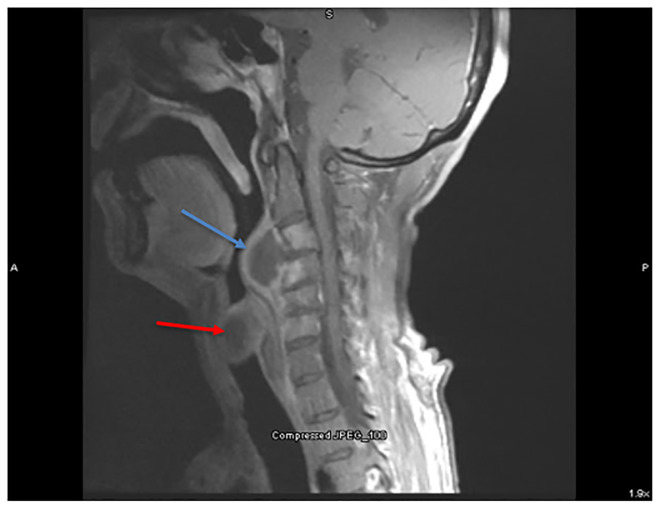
Magnetic resonance imaging of the neck (sagittal view) showing two more enhancing fluid collections, one retropharyngeally abutting the first and second cervical vertebrae (blue arrow), and the other extending into the trachea (red arrow).
